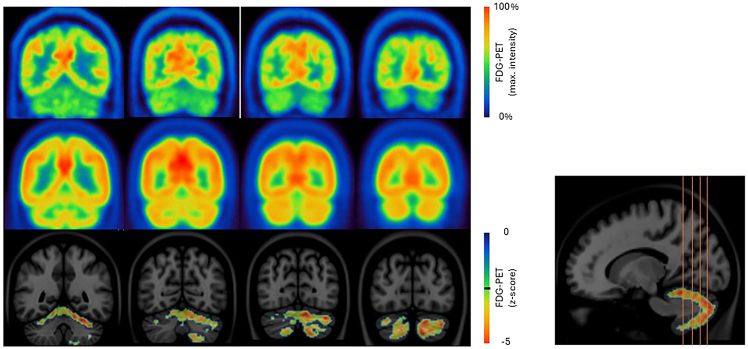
Reduced cerebellar glucose metabolism in index patient 1 on brain fluorodeoxyglucose (FDG)-positron emission tomography (PET) Coronal views of FDG-positron emission tomography (PET) imaging are shown for index patient 1 (top row), compared with a coronal view of a brain FDG-PET template (average of a healthy cohort) (middle row). An overlay of the coronal view of brain FDG-PET and template magnetic resonance imaging (MRI) displaying *Z* scores of index patient 1 versus template (displayed *Z* score: −2,5 to −5) shows a clear reduction in glucose metabolism in the bilateral cerebellar hemispheres (left > right) (lower row). The right panel shows the corresponding layers in sagittal view. See also Figure S7.
